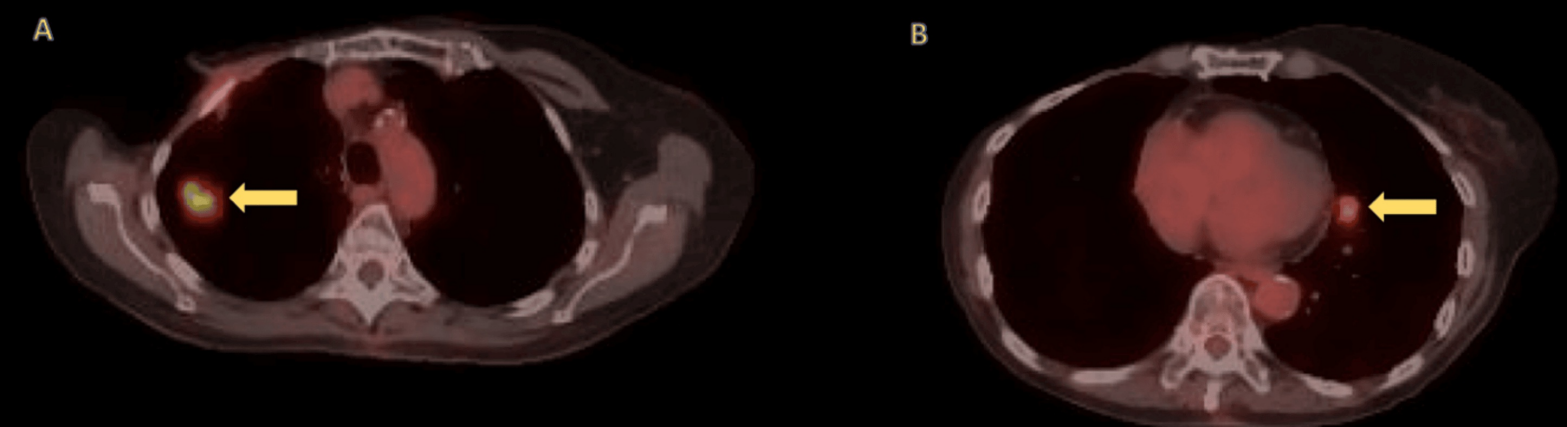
PET/CT imaging demonstrating two FDG-avid pulmonary lesions Panel A: Axial PET/CT showing a spiculated lateral subpleural right upper lobe nodule measuring 11 × 12 mm with intense FDG uptake (SUV max 8.2) (arrow), confirmed as moderately differentiated adenocarcinoma. Panel B: Axial PET/CT showing a juxta-cardiac left lower lobe nodule measuring 10 × 16 mm with moderate FDG uptake (SUV max 3.5) (arrow), confirmed as a typical carcinoid tumor. PET/CT, positron emission tomography/computed tomography; FDG, fluorodeoxyglucose; SUV, standard uptake value
